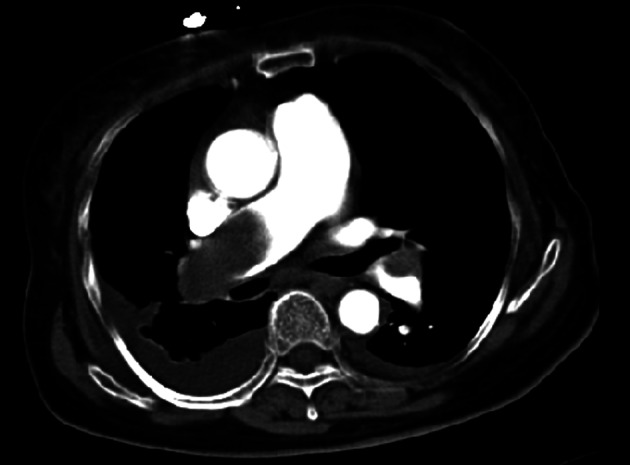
Enhanced chest computed tomography (CT) scan revealing filling defects in the right main pulmonary artery and left lower pulmonary artery branch, as well as bilateral pleural effusion.
